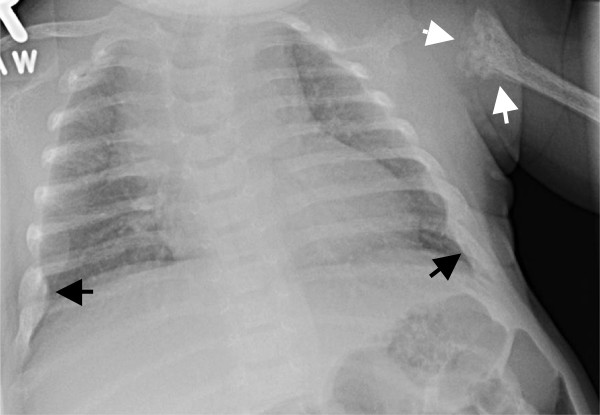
Chest radiograph at presentation. There is fragmentation, mixed sclerosis and lucency of the left upper humeral metaphysis and a small amount of subperiosteal new bone formation extending downward along the medial shaft (white arrows). There are expanded healing rib fractures above both CP angles (black arrows).
